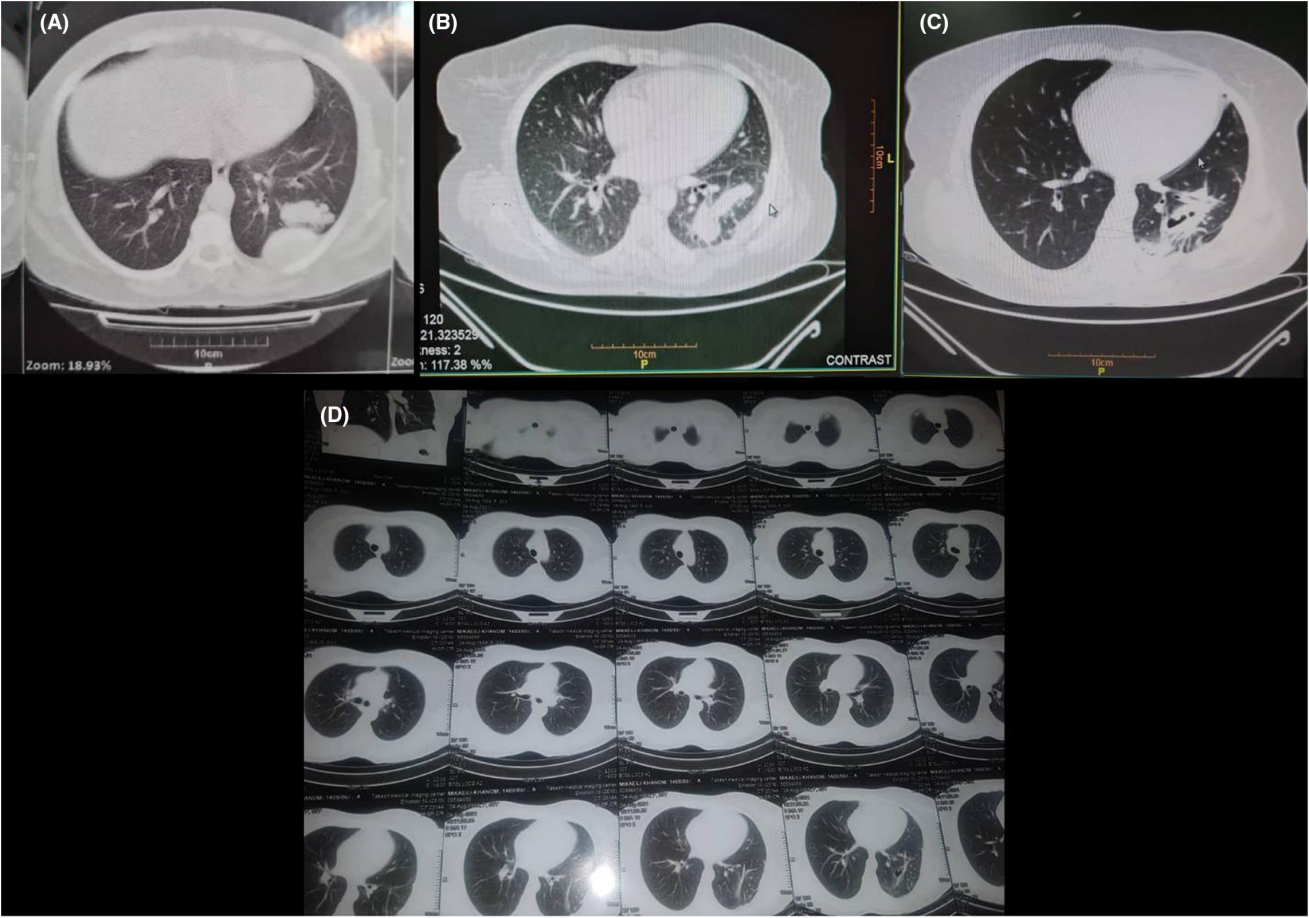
CT scans (A: before surgery; B: After surgery; C: After surgery and amphotericin B therapy; D: 6 months after the treatment and follow‐up).
